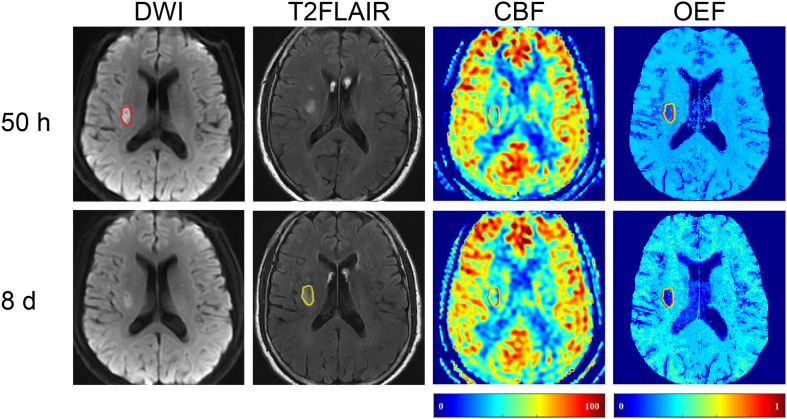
Representative images of a 59-year-old male with left limb weakness. The FI (with a volume of 2.82 ml) on 8-day T2FLAIR (shown in yellow) was equal to the 50-h IDL (2.82 ml) on DWI (shown in red). T2FLAIR, T2 fluid-attenuated inversion recovery; DWI, diffusion-weighted imaging.
